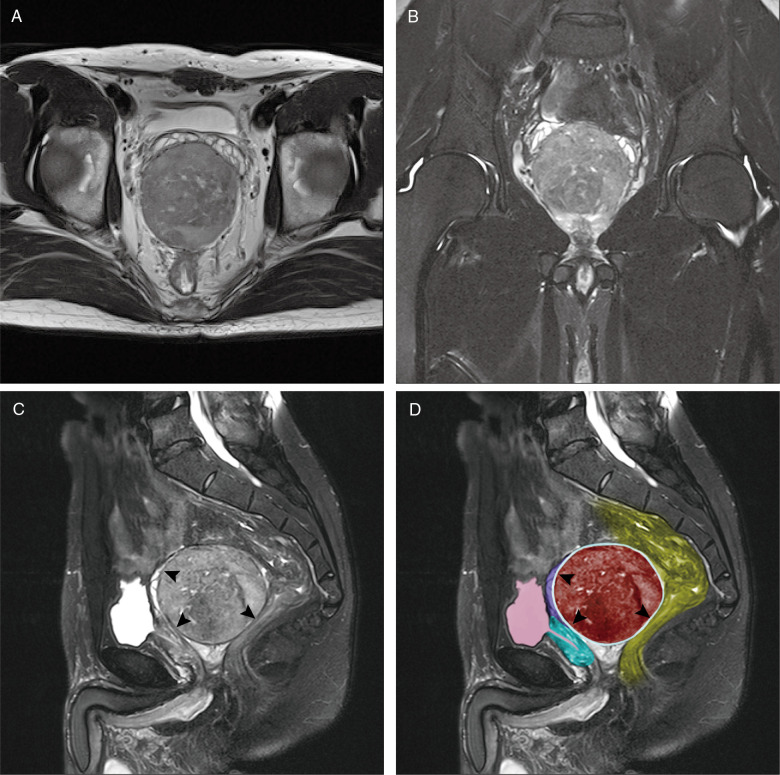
MRI of the leiomyoma of the prostate. MRI T2-weighted axial image (A) and fat-suppressed coronal image (B) shows a round mass in the prostate posterior lobe; fat-suppressed sagittal image (C,D) shows the clear surgical capsule of the tumor (arrow) and shows that normal prostatic glandular tissue and seminal vesicles were compressed anteriorly. The rectum was compressed posteriorly (red: leiomyoma of the prostate; green: prostate; pink: bladder and urethra; yellow: rectum; purple: seminal vesicles; light blue and arrow: surgical capsule of the tumour).
